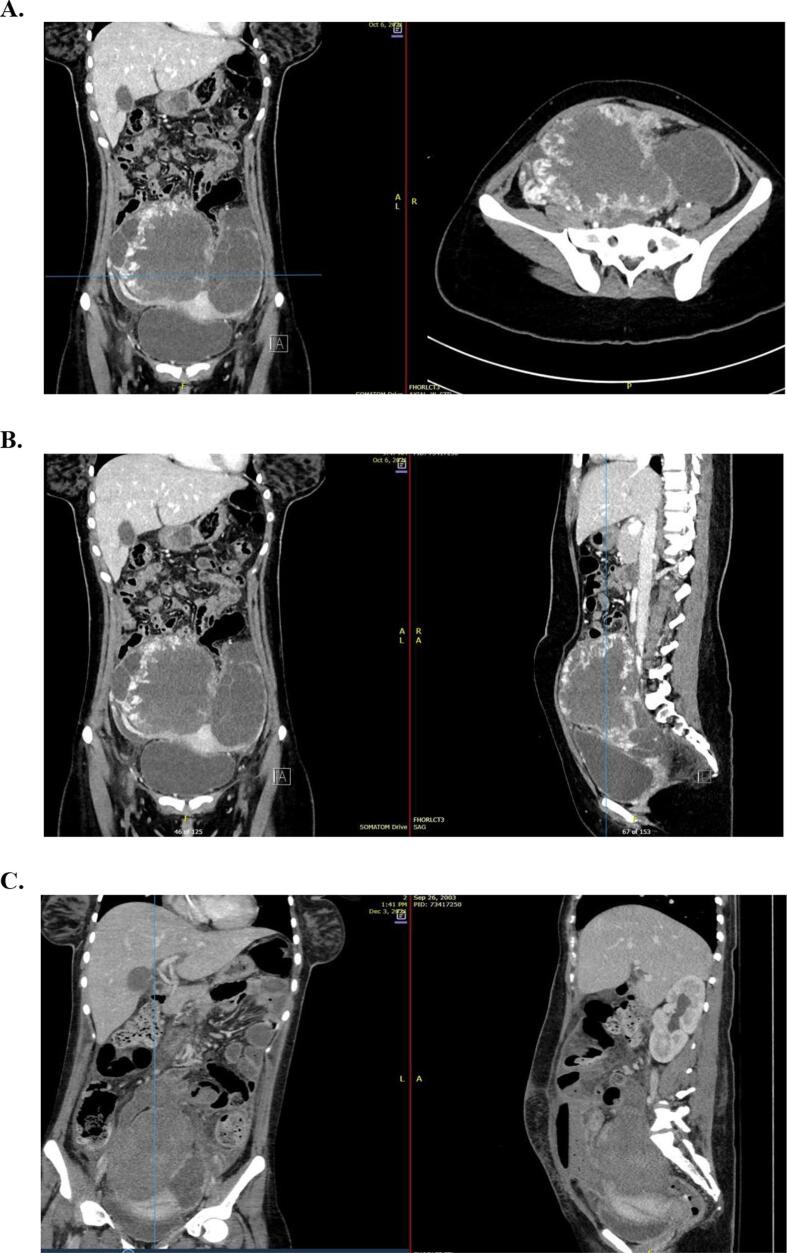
(A) Computed tomography (CT) anterior/posterior (A/P) coronal and transverse. Multiple complex cystic masses in both adnexa measuring up to 15.5 cm and 13.6 cm. (B) CT A/P coronal and sagittal. Multiple complex cystic masses in both adnexa. (C) CT A/P coronal and sagittal. Peritonitis secondary to bowel rupture from tumor invasion to the sigmoid colon.
